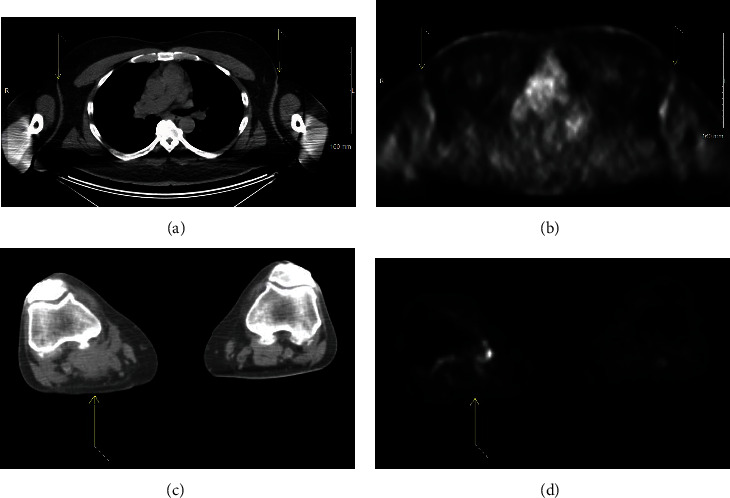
PET/CT imaging approximately 7 months after starting RT. Axial CT of patient axilla (arrows) (a) and corresponding PET image (b). Axial CT of patient's right popliteal mass (arrow) (c) and corresponding PET image (d).
